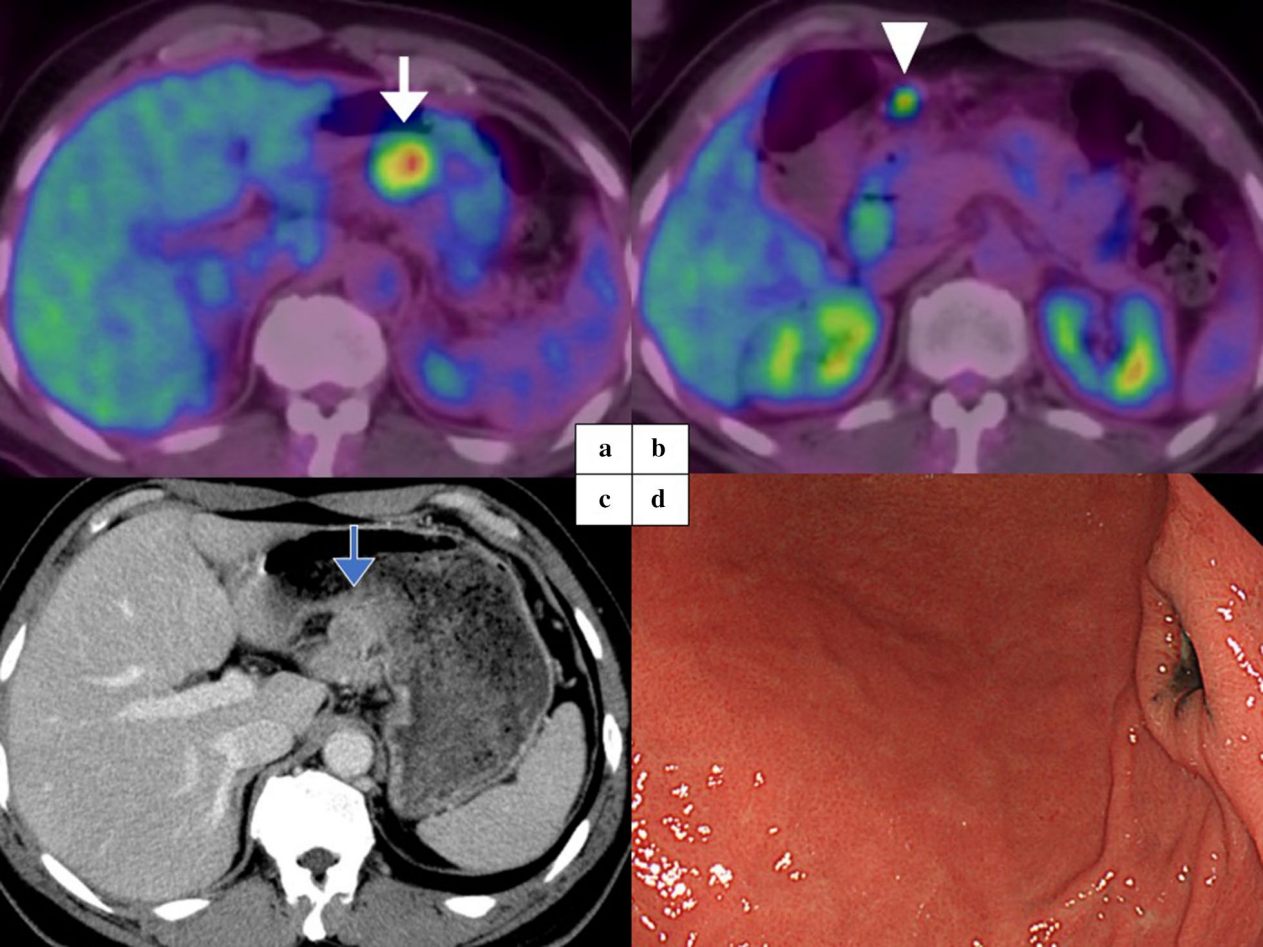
Images of preoperative examinations. a, b The PET–CT images. The white arrow shows uptake around the lymph nodes along the lesser curvature. The white arrowhead shows uptake around subpyloric lymph nodes. c The e-CT image. The blue arrow shows the lymph nodes along the lesser curvature may have invaded the pancreas. d The EGD revealed a 20 mm submucosal tumor-like lesion with a melanotic ulcer on the posterior wall of the gastric angle. PET–CT: fluorodeoxyglucose positron emission tomography and computed tomography, e-CT: enhanced computed tomography, EGD: esophagogastroduodenoscopy
